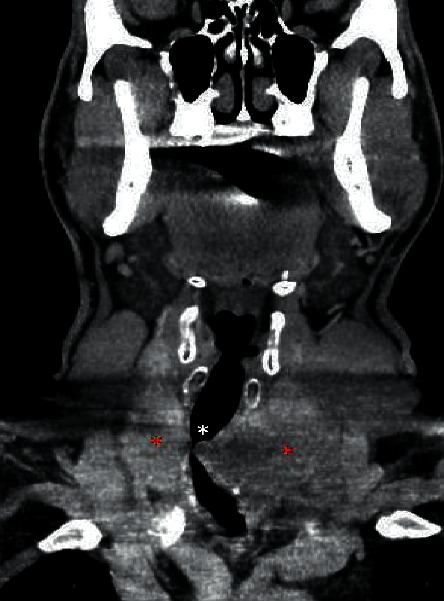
Enhanced coronal computed tomography image demonstrating severe narrowing of the trachea (white asterisk) by bilateral thyroid lobe tumor (red asterisks).
